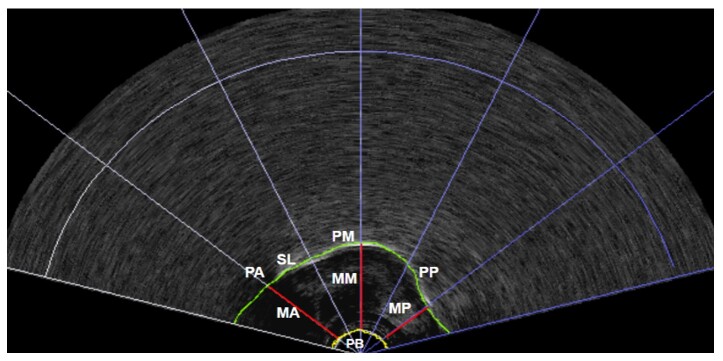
Ultrasound image with an example of the extraction of the anterior, middle, and posterior measures of the tongue
